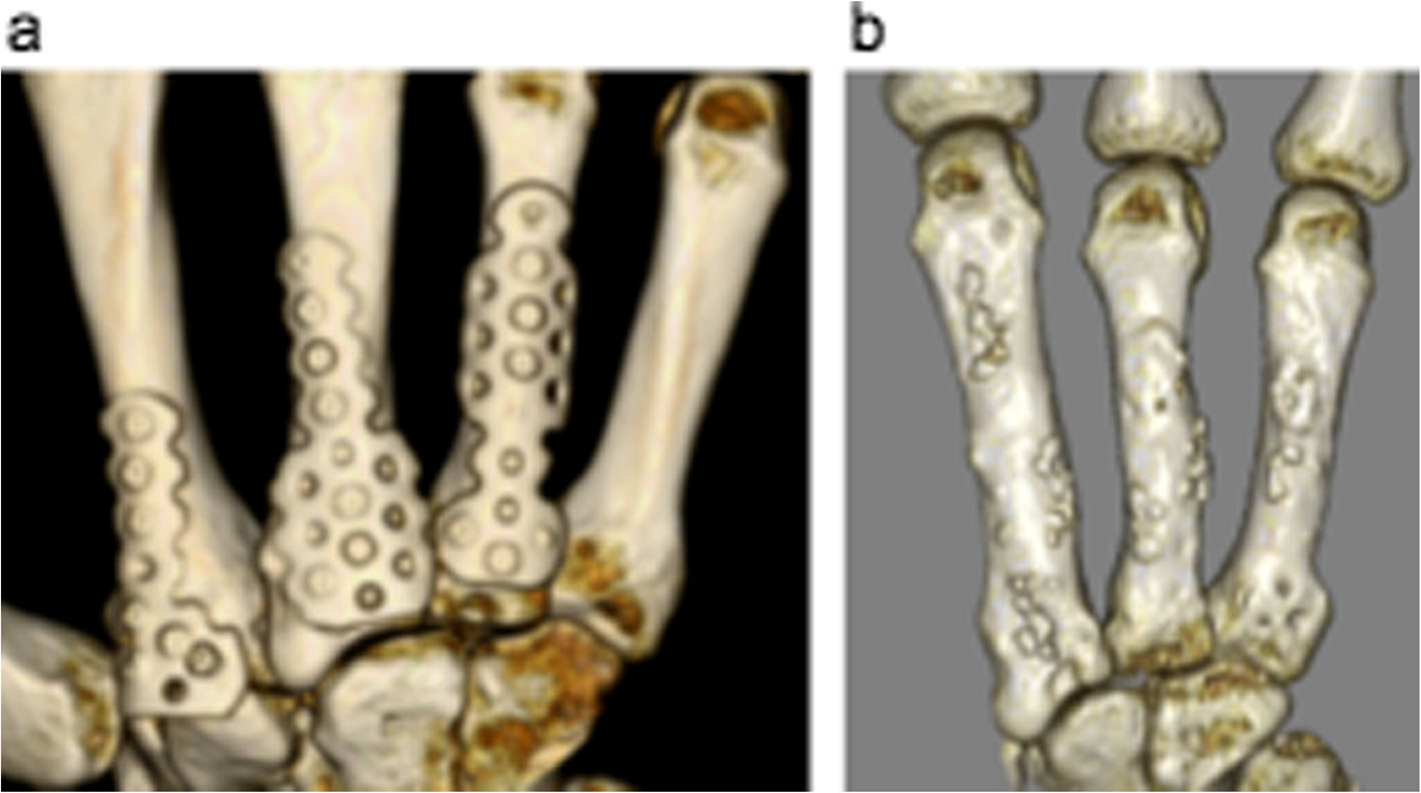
BAP shown on computed tomography scan (a) and is fully bioabsorbable (b). BAP, bioabsorbable plate
